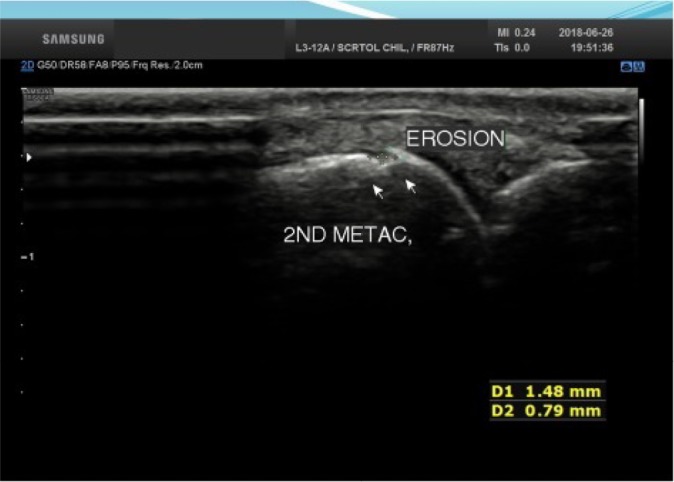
Signs of bone erosion and destruction in ultrasonography.
